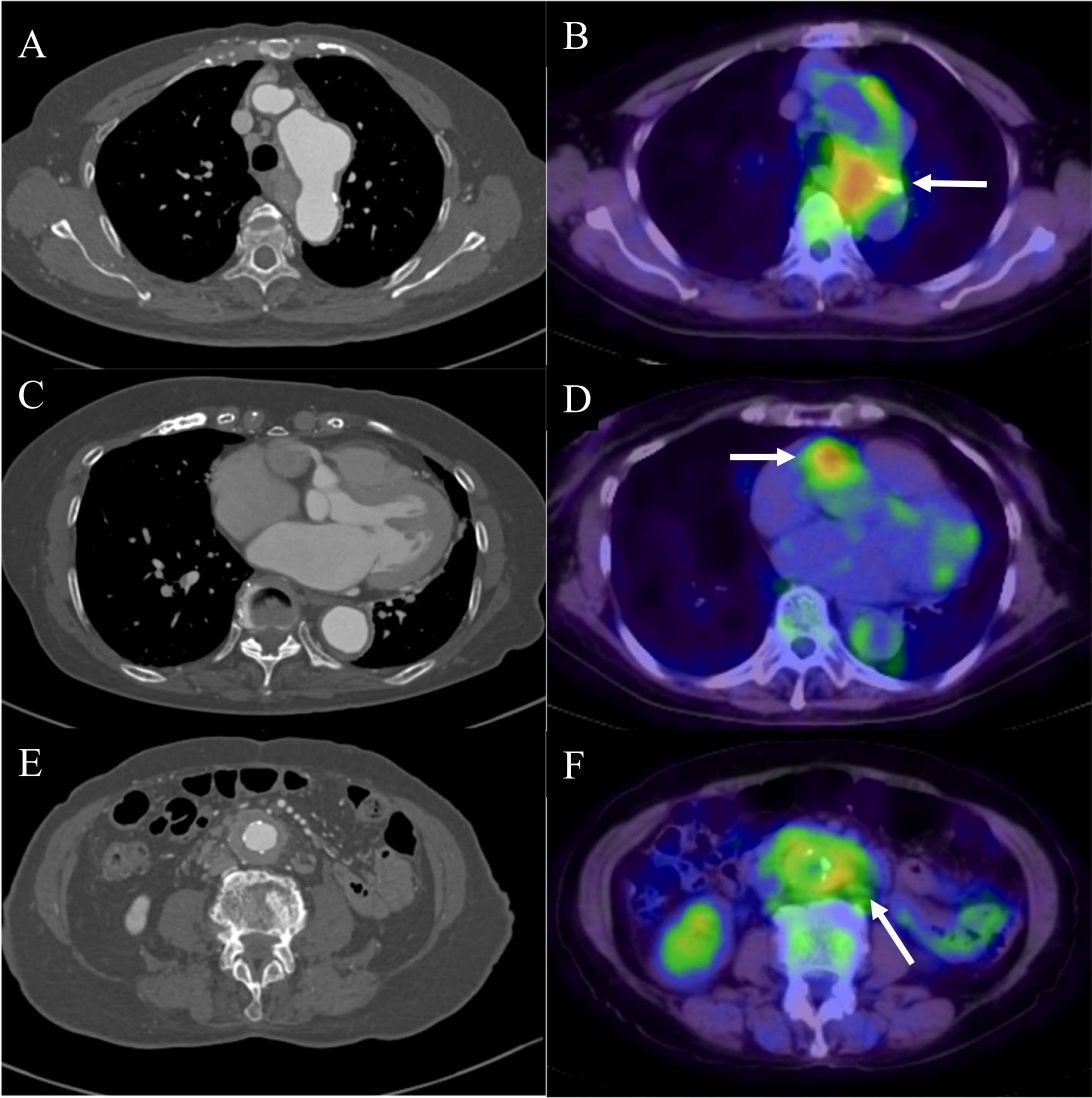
Preoperative enhanced computed tomography (CT) and positron emission tomography (PET)-CT images. Lesions with ^18^F-fluorodeoxyglucose (FDG) uptake are indicated by a white arrow. A, B Images of the thoracic aortic aneurysm (TAA) with a diameter of 50 mm. ^18^F-FDG uptake around the TAA (maximum standardized uptake value [SUVmax]: 5.6). C, D Images of the right coronary artery aneurysm (CAA) with a diameter of 21 mm. ^18^F-FDG uptake around the CAA (SUVmax: 5.1). E, F Images of abdominal aortic aneurysms with a diameter of 30 mm..^18^F-FDG uptake around the abdominal aortic aneurysm (SUVmax: 4.2)
